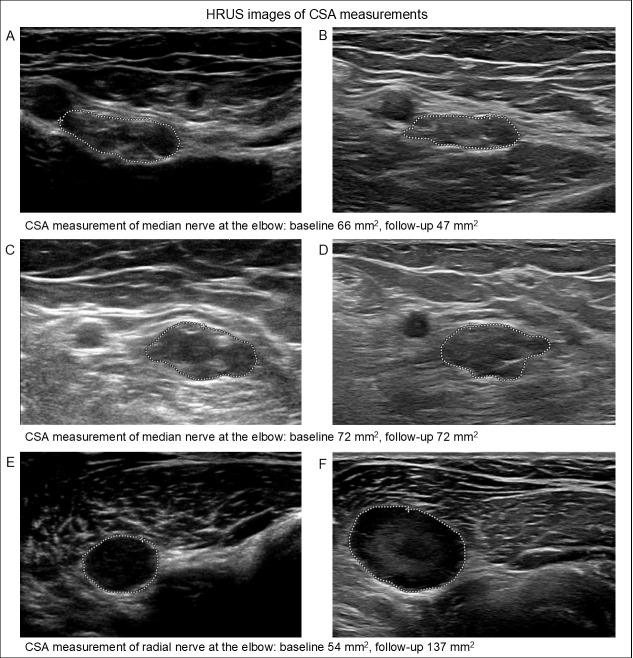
Cross-sectional area (CSA) measurements of different patients at baseline and follow-up. Panels (A,B) show a decrease in CSA, panels (C,D) a stable CSA, and panels (E,F) an increased CSA. HRUS = high-resolution nerve ultrasound.
